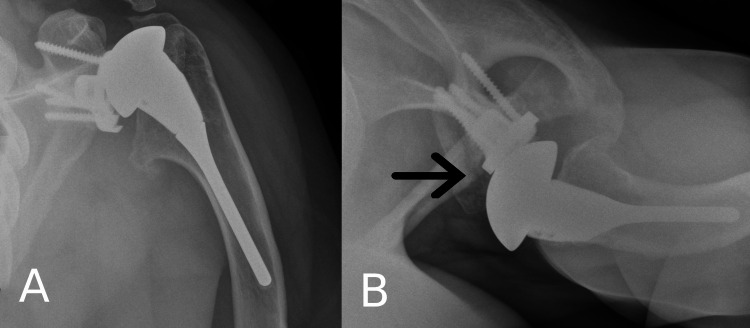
X-rays of the left shoulder revealing implant failure and anterior sublaxation (black arrow) (A) Anteroposterior view; (B) Axial view
